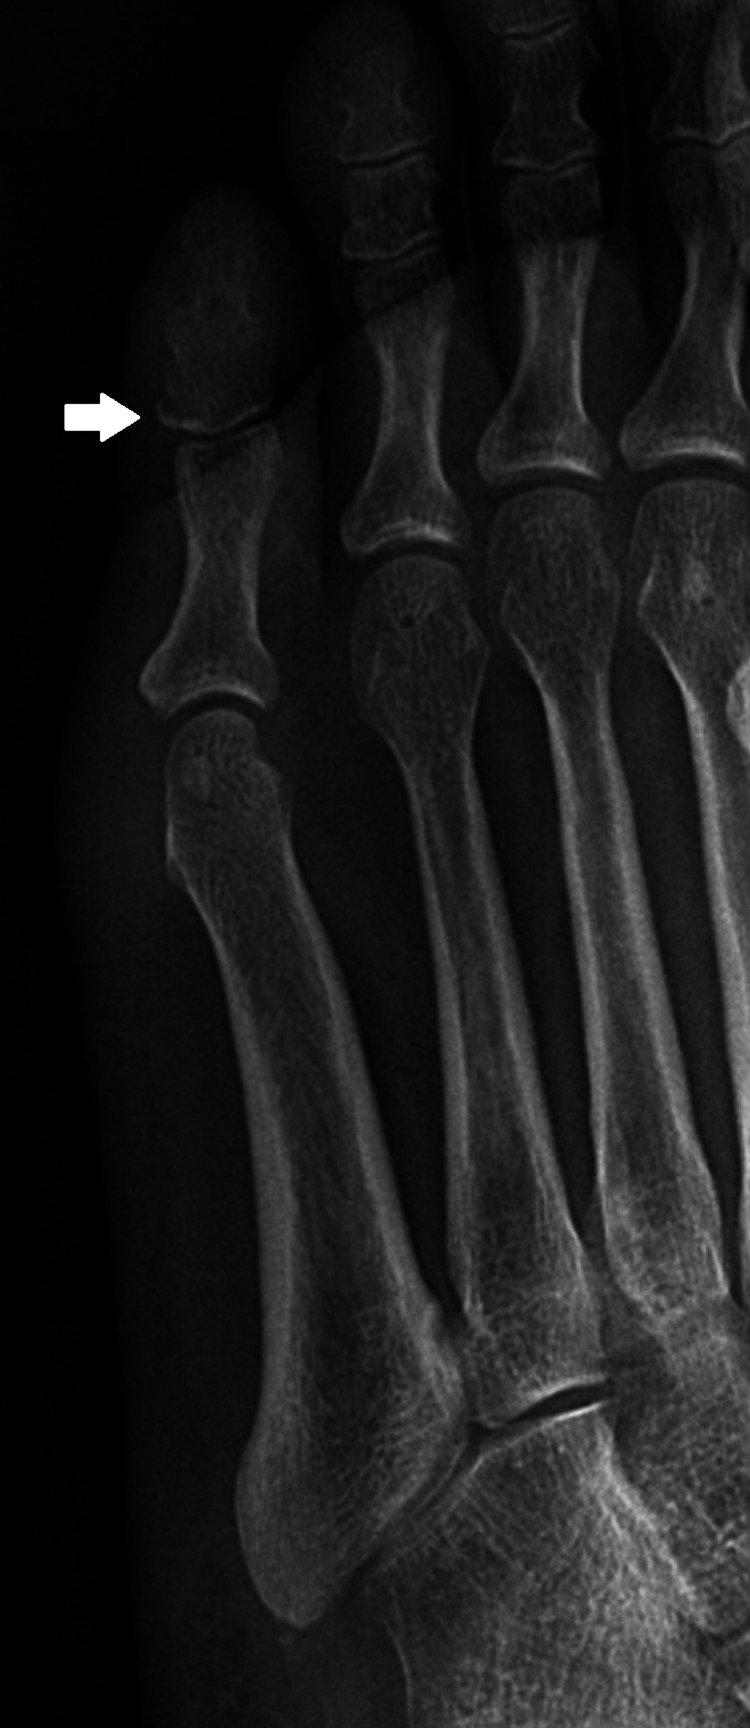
Radiograph of the right foot A non-displaced avulsion fracture of the superolateral aspect of the right distal fifth phalanx
